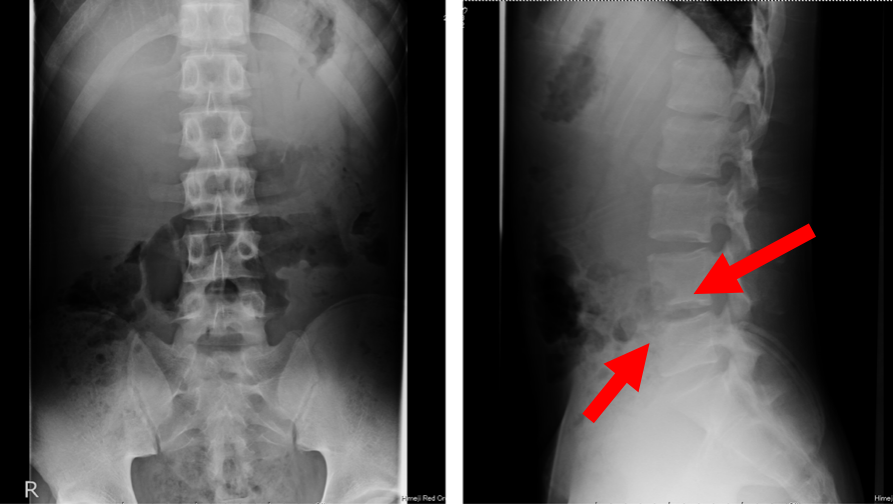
X-ray revealed inhomogeneous appearance of the inferior wall of the L4 and anterior wall of the L5 vertebral bodies
